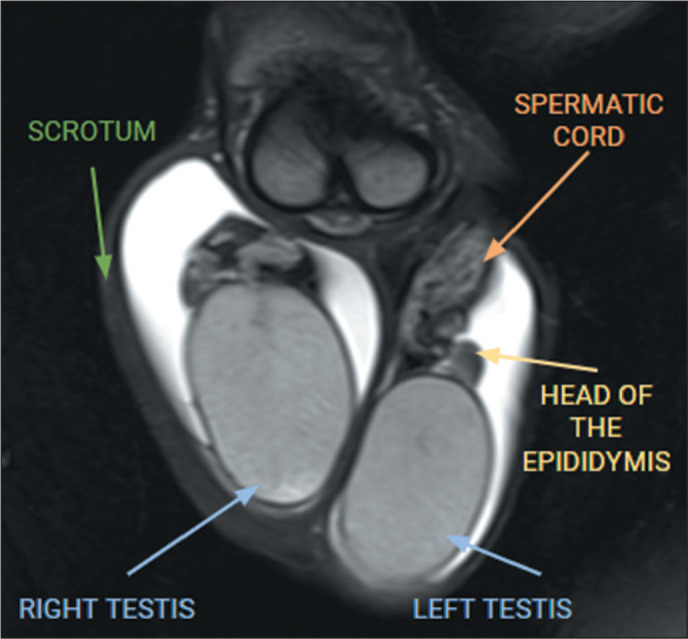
MRI showing the normal anatomy of the scrotum on coronal T2- weighted
